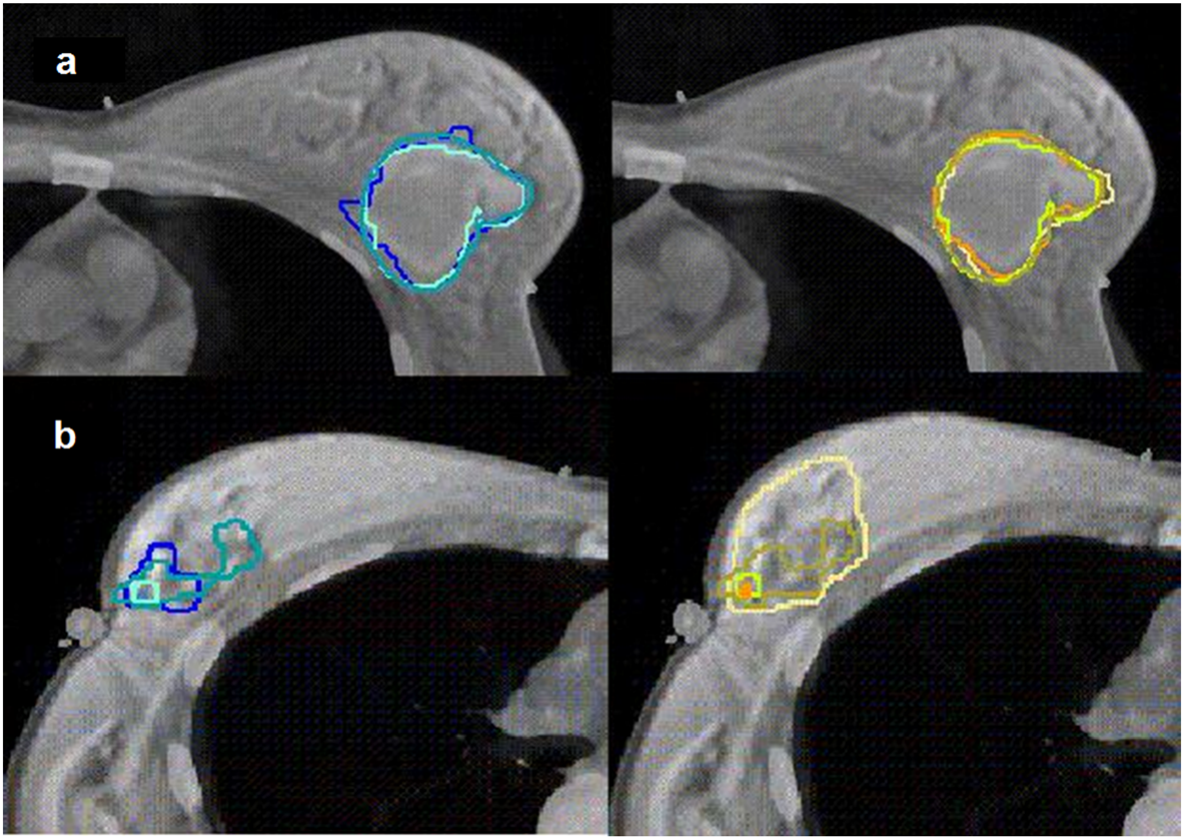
Differences between the 4 observers on co-registered CTMR images; Left: volumes delineated on CT. Right: volumes delineated on CTMR; a. Example of a patient with seroma, a CVS of 5; b. Example of a patient with a CVS of 2.
